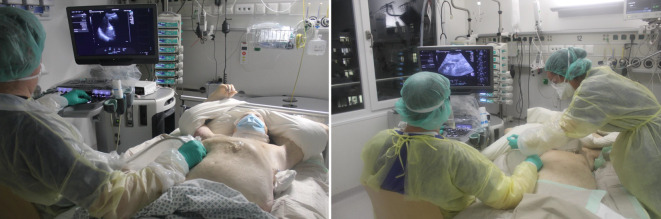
Zwischen Dezember 2021 und Januar 2022 verstarben 6 COVID-19-Patienten auf der Intensivstation. Bei 5 von 6 Fällen stimmten die Angehörigen der US-MIA zu. Diese wurden zwischen 129 und 210 min nach dem Tod durchgeführt, im Durchschnitt nach 162 min. Die Ultraschalluntersuchung dauerte durchschnittlich 13 min (5–16 min), die Punktionen und die Gewebegewinnung dauerten im Durchschnitt 54 min (44–75 min). Die Gesamtdauer der US-MIA lag somit im Durchschnitt bei 67 min, das postmortale Intervall betrug durchschnittlich 215 min. Insgesamt wurden 318 Multiorganproben gewonnen und 123 FFPE-Biopsien hinsichtlich ihrer Qualität und Diagnose bewertet. Von den Gewebeproben enthielten 96 % organspezifisches, diagnostisch verwertbares Gewebe. Der Erhaltungszustand der Gewebe war exzellent, mit lichtmikroskopisch und exemplarisch auch elektronenmikroskopisch nachgewiesenen optimal erhaltenen zellulären und subzellulären Strukturen (Abb. 2). Die durchschnittliche Biopsielänge betrug 11 mm (4–25 mm). In allen Lungenproben wurden lichtmikroskopisch typische SARS-CoV-2-induzierte Veränderungen in unterschiedlicher Ausprägung beobachtet, darunter ein diffuser Alveolarschaden in verschiedenen Stadien, Metaplasien, Mikrothromben, Endothelialitis und in einem Fall eine *Aspergillus*-Superinfektion. Extrapulmonale Befunde umfassten schockbedingte Veränderungen, insbesondere in der Leber und Niere, sowie verschiedene Grunderkrankungen. Die postmortale Ultraschalluntersuchung enthüllte pathologische Befunde an verschiedenen Organen, die vor dem Tod des Patienten nicht bekannt waren, darunter eine Pankreaszyste mit pankreaner intraepithelialer Neoplasie 1a, ein Niereninfarkt bei *Aspergillus*-Sepsis, ein frisches retroperitoneales Hämatom und eine sich organisierende Beinvenenthrombose. In Bezug auf die infektionshygienische Sicherheit des Verfahrens konnten wir erneut bestätigen [14], dass die US-MIA nicht zur Kontamination der Umgebung führt. Bei 54 von 55 auf SARS-CoV‑2 getesteten Abstrichen wurde keine virale SARS-CoV-2-RNA nachgewiesen. Lediglich in einem Abstrich (Ultraschallsonde mit unmittelbarem Kontakt zur Nadeleinstichstelle) konnte SARS-CoV-2-RNA nachgewiesen werden (CT/ORF 37).
